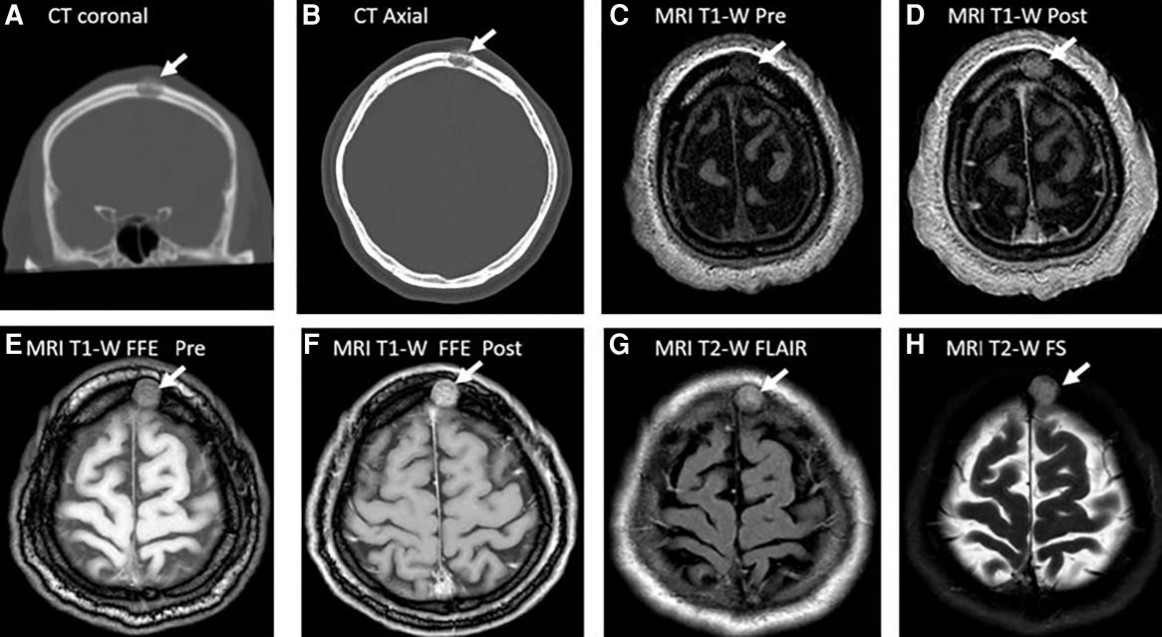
CT and MRI scans of a high-risk patient (case 1) with an intraosseous hemangioma (arrow). (A) CT scan without contrast, coronal image, with a 1.6 × 2 × 1.4-cm (TR × AP × CC) lytic lesion in the left superior frontal calvarium with interruption of the inner and out bone tables. (B) CT scan without contrast, axial image, showing the lytic lesion. (C) MRI scan, T1-weighted (T1-W) pregadolinium, axial image. (D) MRI scan, T1-weighted postgadolinium, axial image, showing an enhancing lesion with heterogeneous enhancement. (E) MRI scan, T1-weighted fast field echo (FFE) pregadolinium, axial image, showing a more obvious lesion with a fat suppressed signal. (F) MRI scan, T1-weighted FFE postgadolinium, axial image with improved visualization of the lesion with suppression of fat signal. (G) MRI scan, T2-weighted (T2-W) fluid attenuated inversion recovery (FLAIR), axial image, suppresses CSF signal showing a hyperintense lesion. (H) MRI scan, T2-weighted fat-suppressed (FS), axial image further improves lesion detection with suppression of the hyperintense fat signal.
